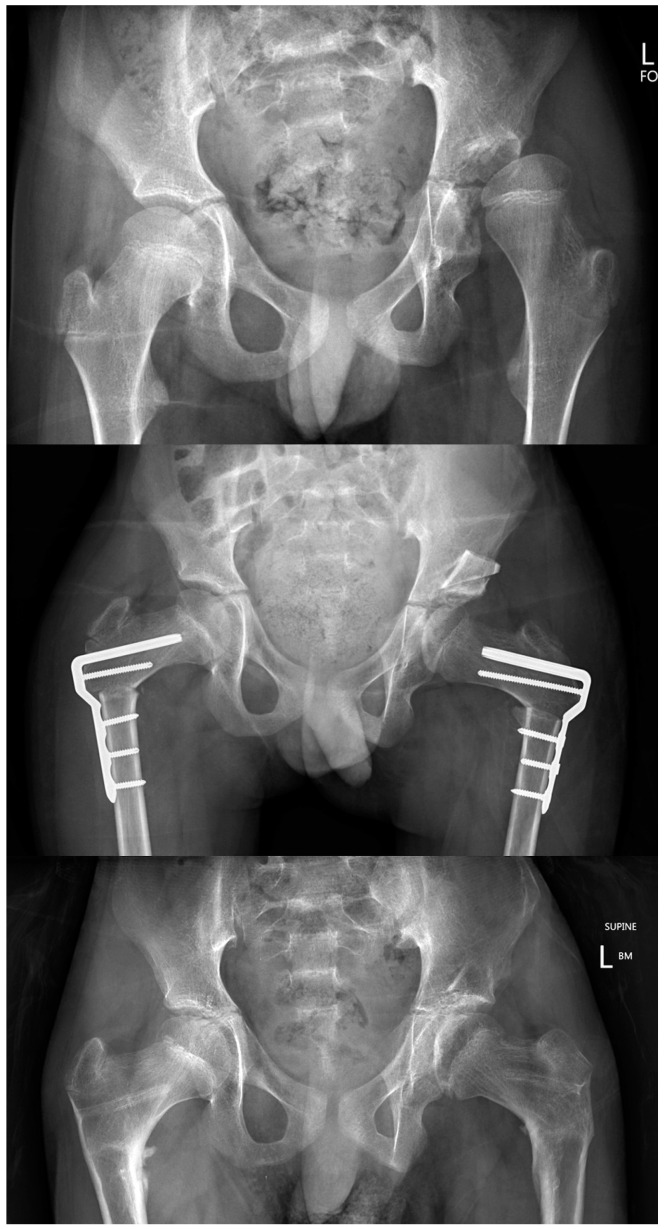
Bilateral surgery for unilateral CPHD. Copyright Kerr Graham, RCH Melbourne. These are the radiographs of a boy aged 10 years, GMFCS IV with clinical and radiographic unilateral (left) CPHD. The top image is immediately pre-operative. The middle image is 7 days after bilateral APR, bilateral VDRO and left San Diego Acetabuloplasty. The bottom image is at 2-year follow-up, after removal of implants. Bilateral surgery gives the best opportunity for symmetry and durability of outcome with marginal increases in surgical adverse events and morbidity.
